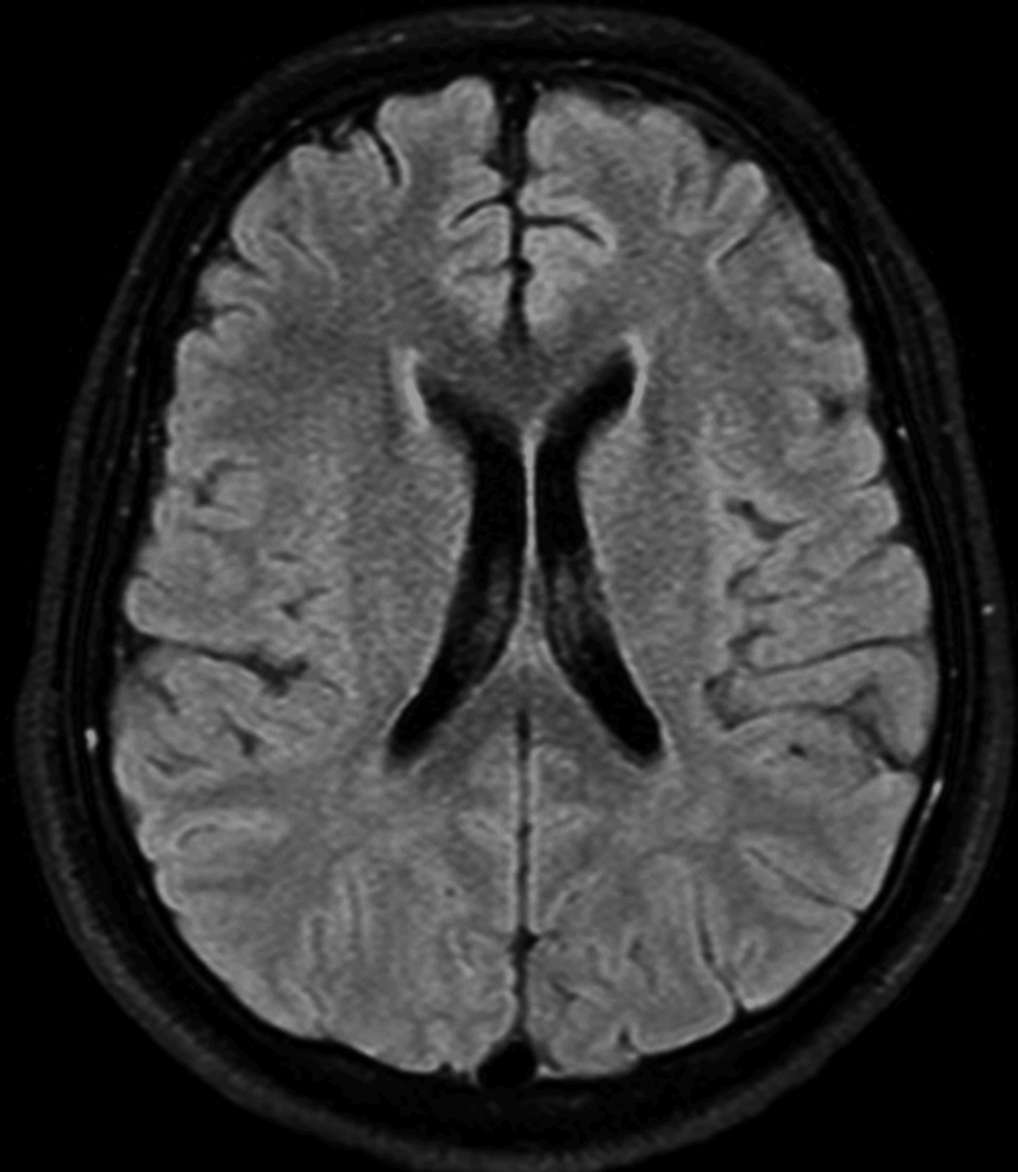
Normal axial brain MRI in fluid-attenuated inversion recovery (FLAIR) sequence. The image shows normal anatomical structures without any signs of abnormal signal changes in the patient.
